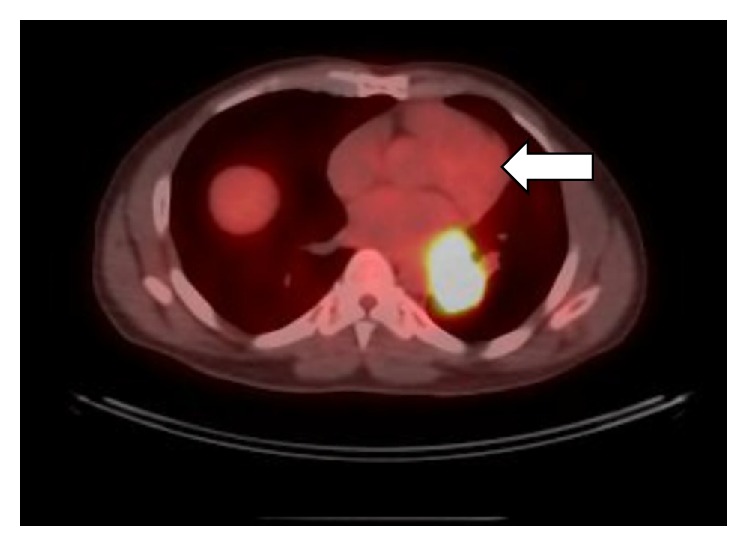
PET CT showing left lobe mass.
